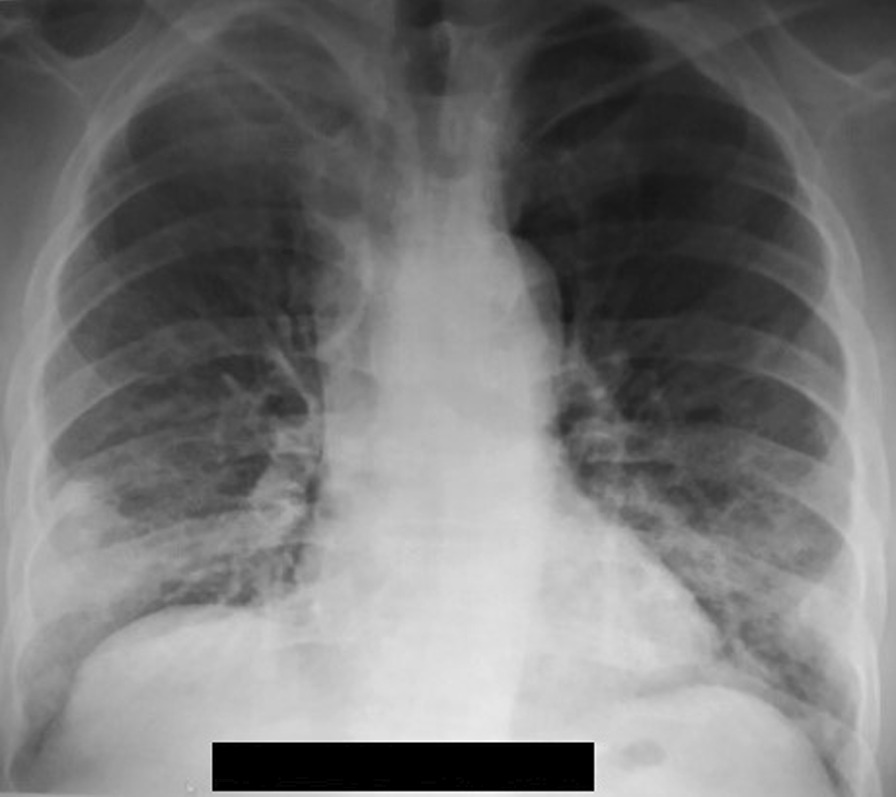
X-ray of case 2 showing bilateral ill-defined airspace consolidations involving mostly lower zones with peripheral distribution
